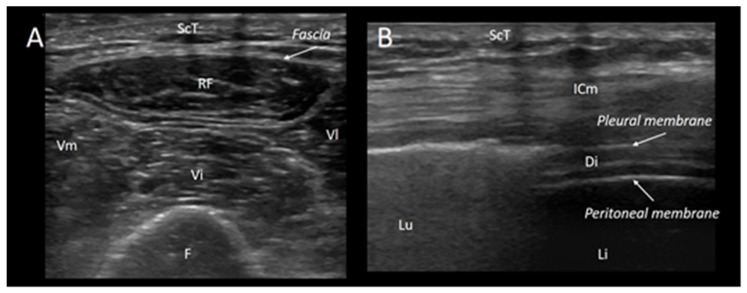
Normal features of diaphragm and quadriceps muscle. The figure shows the features that can be seen with skeletal muscle ultrasound. Left Panel (A): a transverse scan of the thigh allows for the visualization of the quadriceps muscle, which is composed of the rectus femoris (RF), the vastus lateralis (Vl), the vastus medialis (Vm), and the vastus intermedius (Vi); the hyperechoic, curvilinear image on the lower part of the figure, with the anechoic shadow is the femur (F), while on the upper part of the image, the subcutaneous tissue is shown (ScT). Right panel (B): a longitudinal scan of the chest wall at the zone of apposition allows for the visualization of the diaphragm at the zone of apposition (i.e., the area of attachment between the diaphragm directly behind the inner aspect of the lower chest wall and rib cage). Lu: lung; ScT: subcutaneous tissue; ICm: intercostal muscles; Li: liver; and Di: diaphragm.
